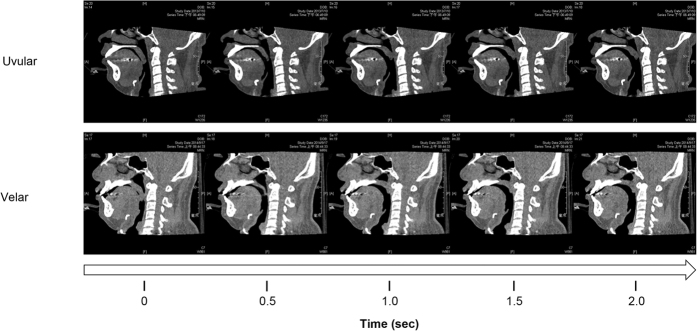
Types of anterior-posterior palatal obstruction. Two types (uvular and velar) of anterior-posterior palatal obstruction were classified in drug-induced sleep computed tomography.
